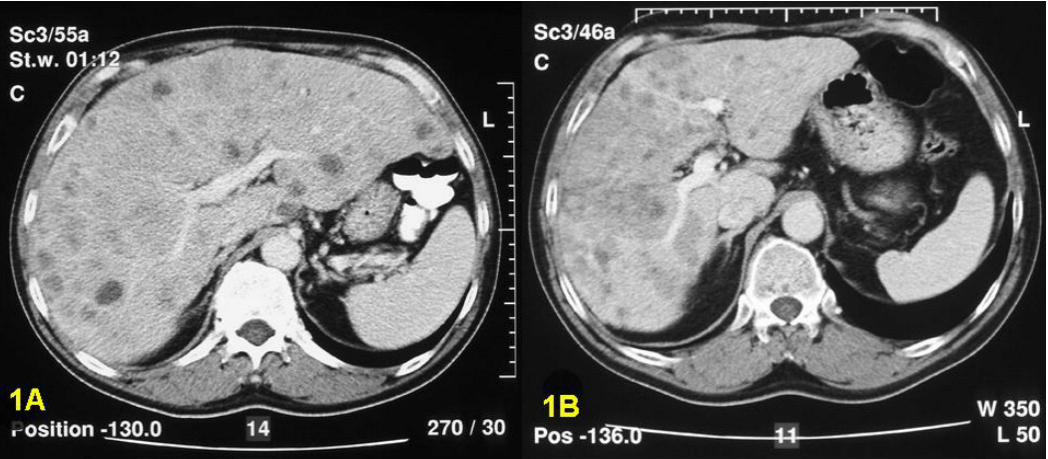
In patient 12 the initial CT-scan (figure 1A) of the liver shows multiple small nodules in both liver lobes. After HAE (figure 1B) the metastases are confluent and the total volume of the liver has decreased remarkably. Symptoms improved and a biochemical response was obvious.
